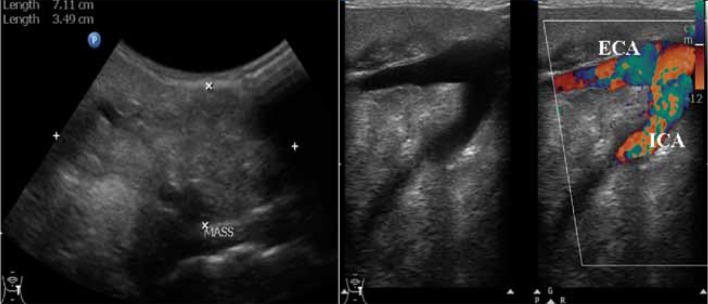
Ultrasound Shows a Well-Defined Heterogeneous Isoechoic Hypovascular Mass Lesion in Relation to the Left Parapharyngeal Region Encasing and Splaying the Carotid Vessels With Central Calcifications.
